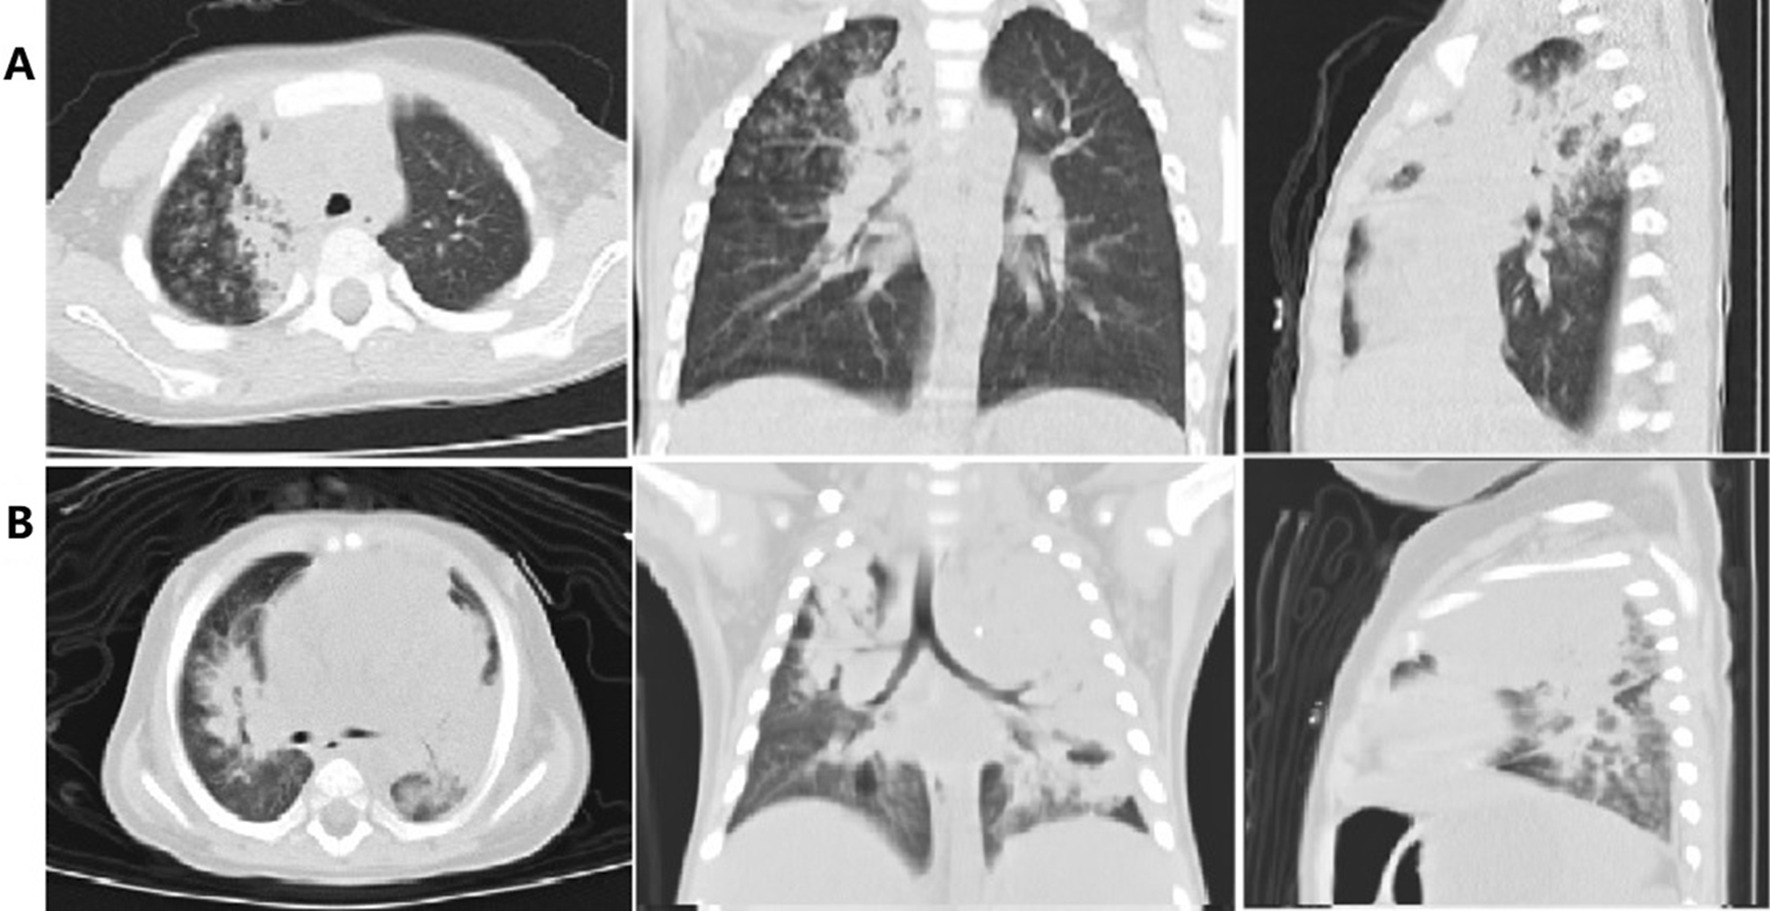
Imaging features of patients with mild and severe adenovirus pneumonia. a High-resolution computed tomography (CT) of the chest on the day of admission revealing diffuse infiltration and few areas of consolidation in the right upper in a child with mild adenovirus pneumonia. b High-resolution CT of the chest on the day of admission revealing areas of airspace consolidation in the left lobes and lingual lobe in a child with severe adenovirus pneumonia
